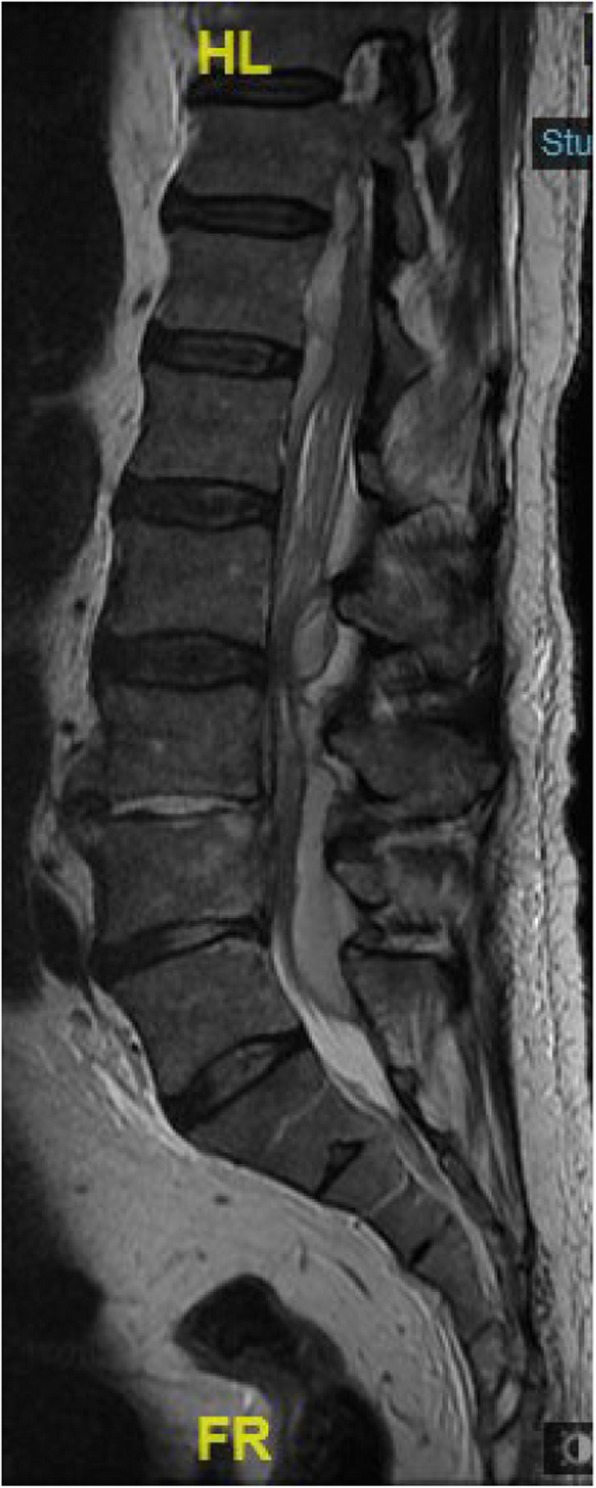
Spinal epidural abscess as seen on magnetic resonance imaging of the lumbar spine on day 19 post-endoscopic retrograde cholangiopancreatography
